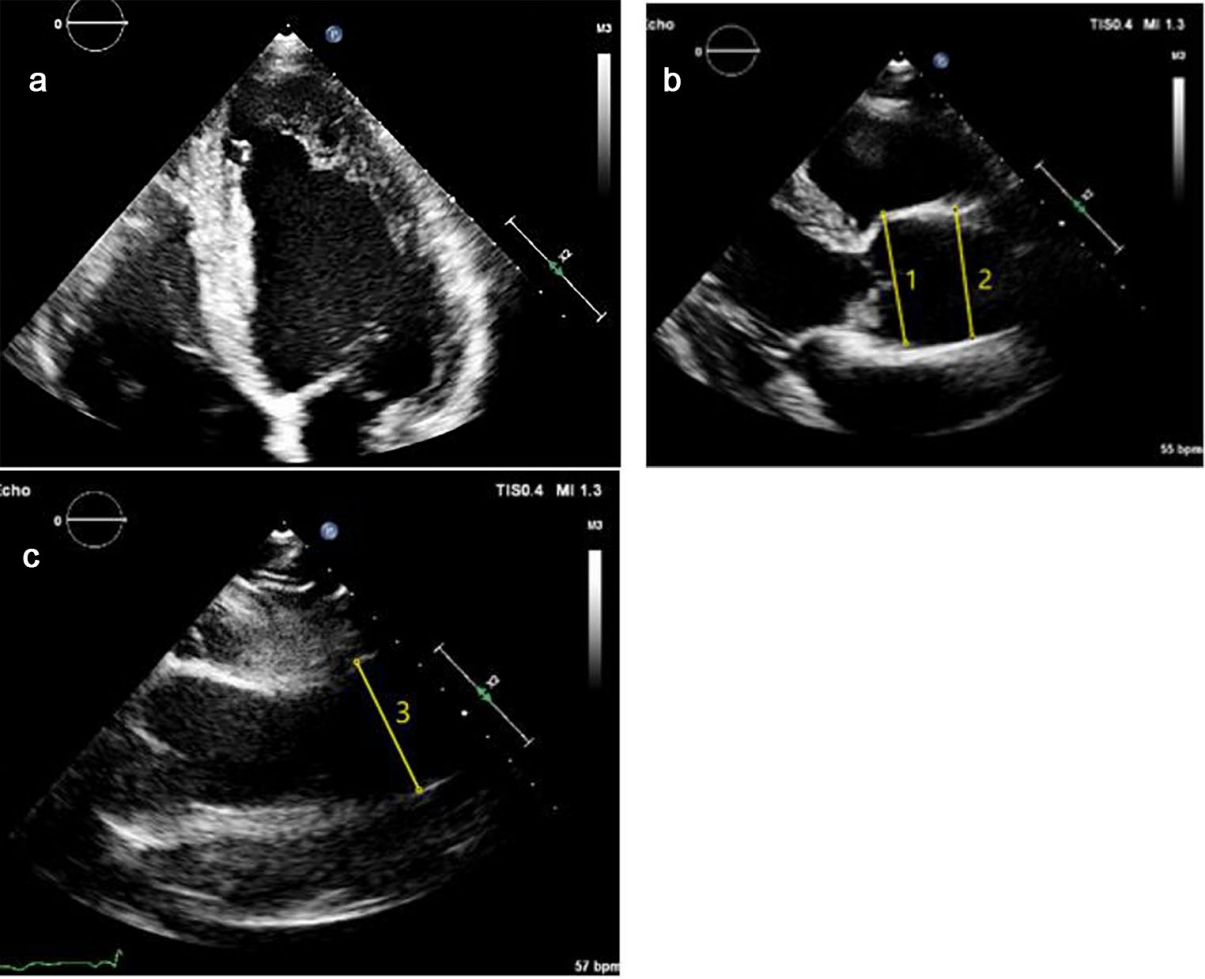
a, b and c: Measuring technique of the aortic dimensions. Transthoracic echocardiogram images in parasternal long axis view of a 54-year-old male patient with noncompaction cardiomyopathy (NCCM). He had non-sustained ventricular tachycardia at presentation. Figure 1a shows the hypertrabeculation of the left ventricular myocardial walls. Figure 1b presents the measurement of the sinuses of valsalva (48 mm) (1), as well as the sinotubular junction (42 mm) (2). Figure 1c presents the measurement of the ascending aortic diameter, which was 43 mm. The patient had no known familial NCCM and there were no genetic variants found
